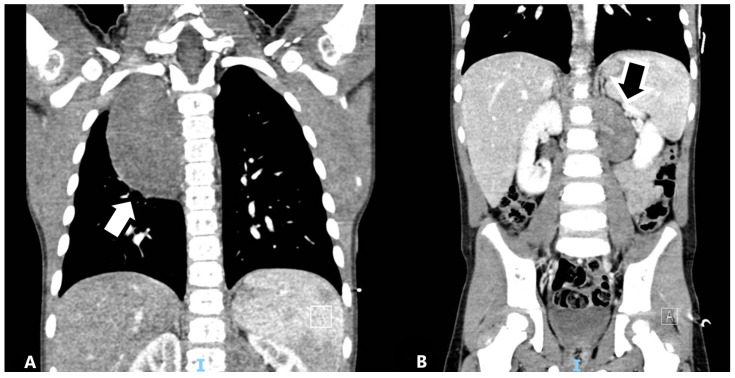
Computed tomography (CT) scan at diagnosis. The patient was a 4-year-old male with a delay in the achievement of normal developmental milestones as well as scoliosis. Difficulty walking was noted, so an X-ray of the spine was performed with the incidental finding of a mass located in the posterior mediastinum, making second-level imaging such as CT necessary. The images show the presence of a right paravertebral mediastinal mass (A) measuring 5.5 × 4.5 × 8 cm (corresponding to a volume of 99 mL), oval-shaped with homogeneous enhancement, and showed the presence of a second bilobed mass located at the left abdominal paravertebral level measuring 4.5 × 3.5 × 5.5 cm (corresponding to a volume of 43 mL) with similar CT features (B). At the thoracic level, the mass was in contact with the right main bronchus and the terminal part of the azygos vein, with no apparent infiltration of adjacent organs and structures; the abdominal lesion reached the kidney ileus with strict contact with both renal vein and artery.
